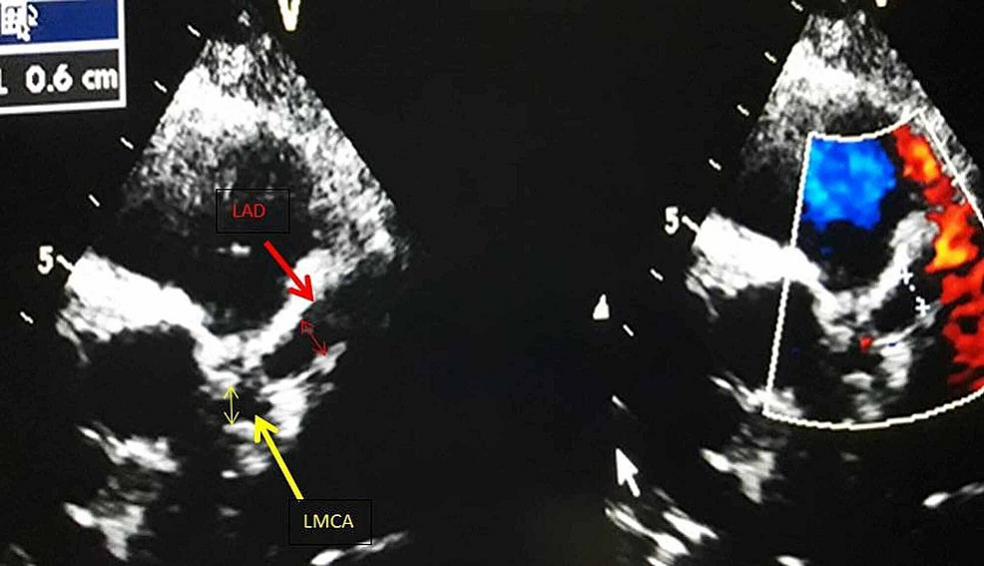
Echocardiography: parasternal short axis view showing left main coronary artery (LMCA) 6mm, left anterior descending artery (LAD) 6.2mm
